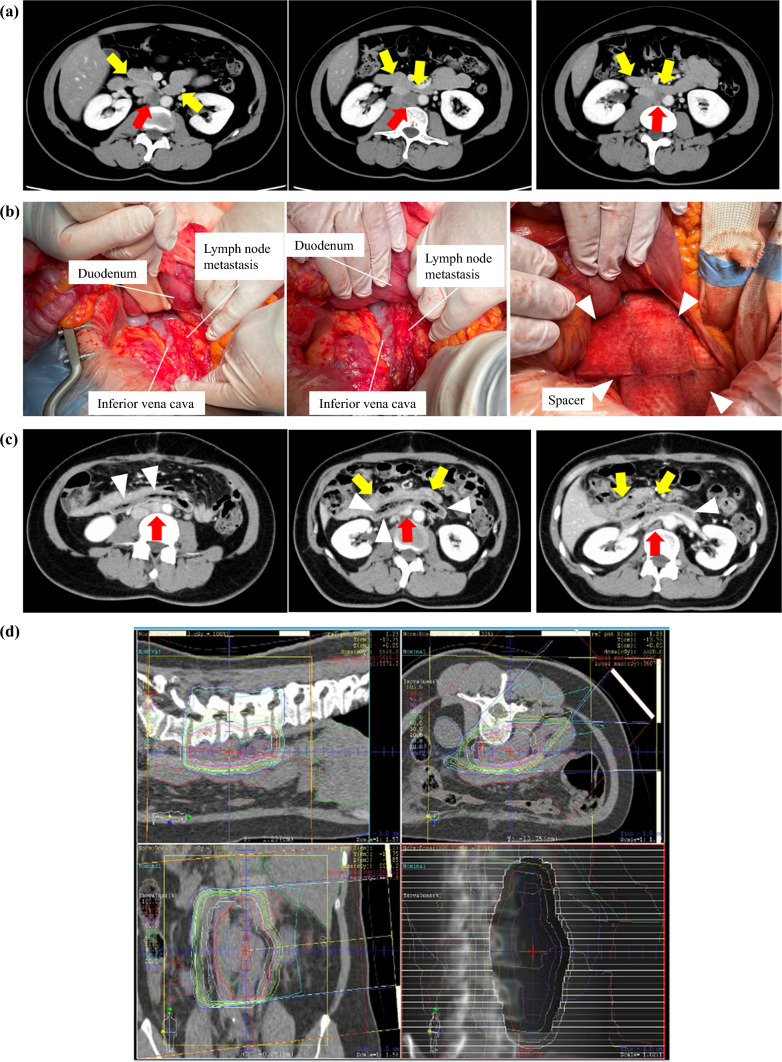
Representative case undergoing space-making particle therapy for lymph node metastasis from uterine cancer in a paraaortic lesion; a abdominal computed tomography before spacer placement surgery showing that the lymph node metastasis (red arrow) between the abdominal aorta and inferior vena cava abuts the duodenum (yellow arrow); b intraoperative findings; after Kocher’s maneuver, the lymph node metastasis located on the left side of the inferior vena cava was dissected from the duodenum; a polyglycolic acid spacer (white arrowhead) was placed between the tumor and the duodenum; c postoperative abdominal computed tomography revealing that the polyglycolic acid spacer (white arrowhead) kept sufficient space between the lymph node metastasis (red arrow) and the duodenum (yellow arrow); d carbon ion therapy with 55.2 gray (relative biological effectiveness) in 12 fractions was delivered; the V95% (volume irradiated with 95% of the treatment planning dose) of the gross tumor volume and clinical target volume were 100.0% and 99.4%, respectively; the D95% (dose intensity covering 95% of the target volume) of the gross tumor volume/planned dose and clinical target volume/planned dose were 98.9% and 98.8%, respectively; D2cc of the duodenum was 2.6 gray (relative biological effectiveness)
